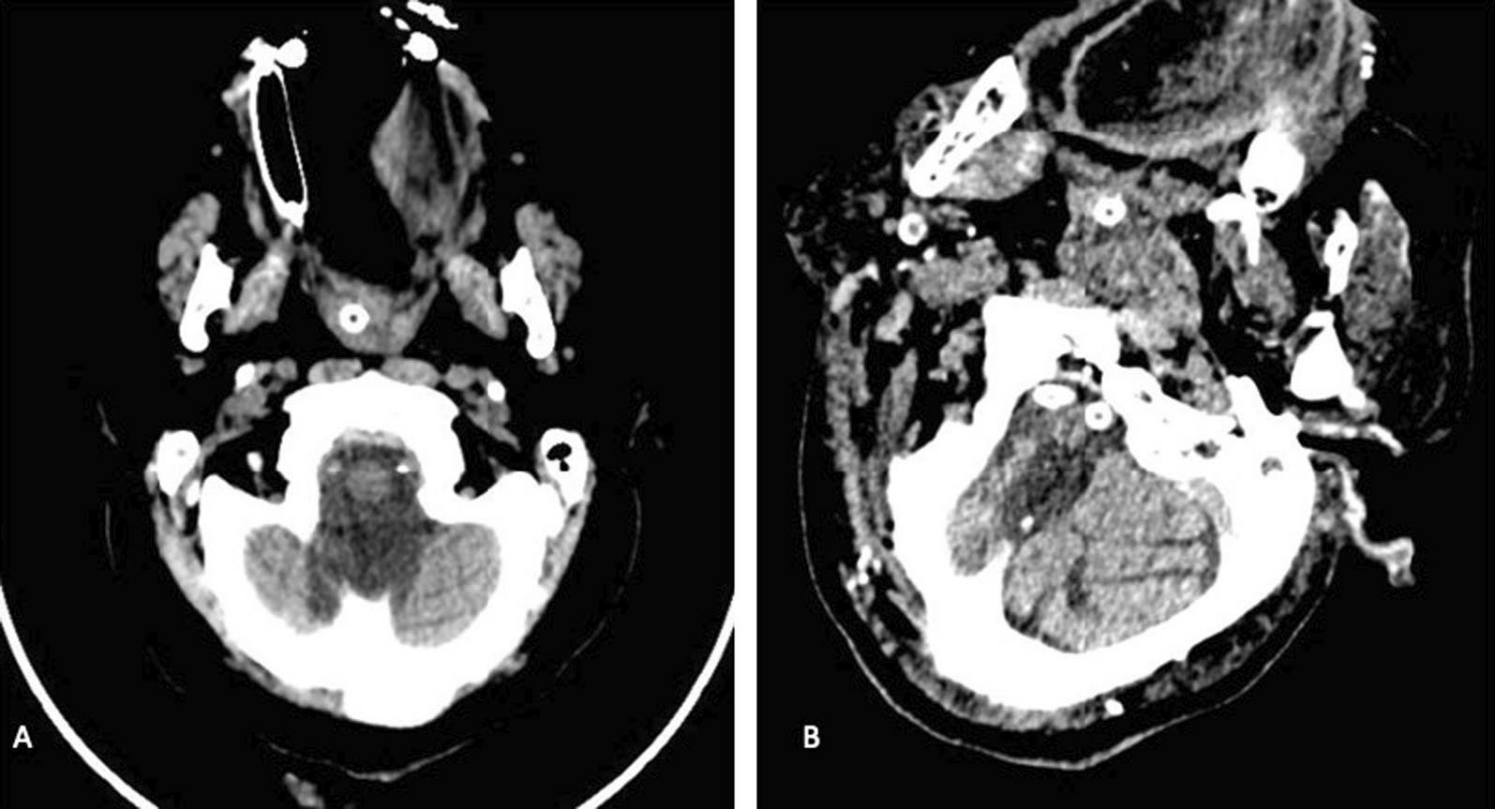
Two different patients showing posterior circulation calcification. A A 46-year-old male patient with a history of posterior stroke, non-enhanced CT brain of posterior fossa demonstrating dense calcifying in the left vertebral artery. B A 55-year-old male patient with a history of stroke, non-enhanced CT brain of posterior fossa showing a dense calcification in bilateral vertebral arteries
